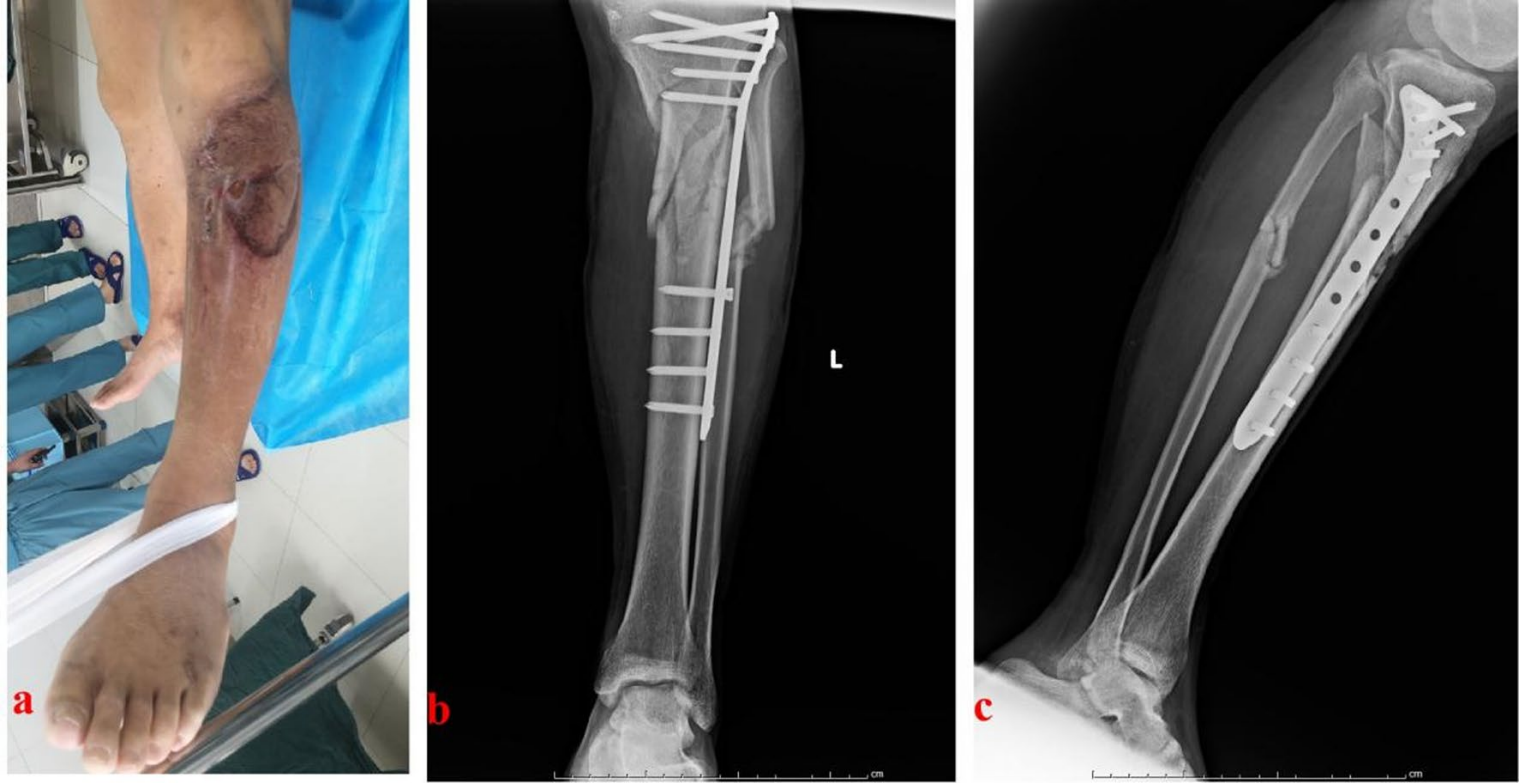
A 43-year-old male suffered from proximal tibial osteomyelitis. (a) A sinus could be found from the injured limb; (b) and (c) The initial X-ray films of the injured lower leg.
